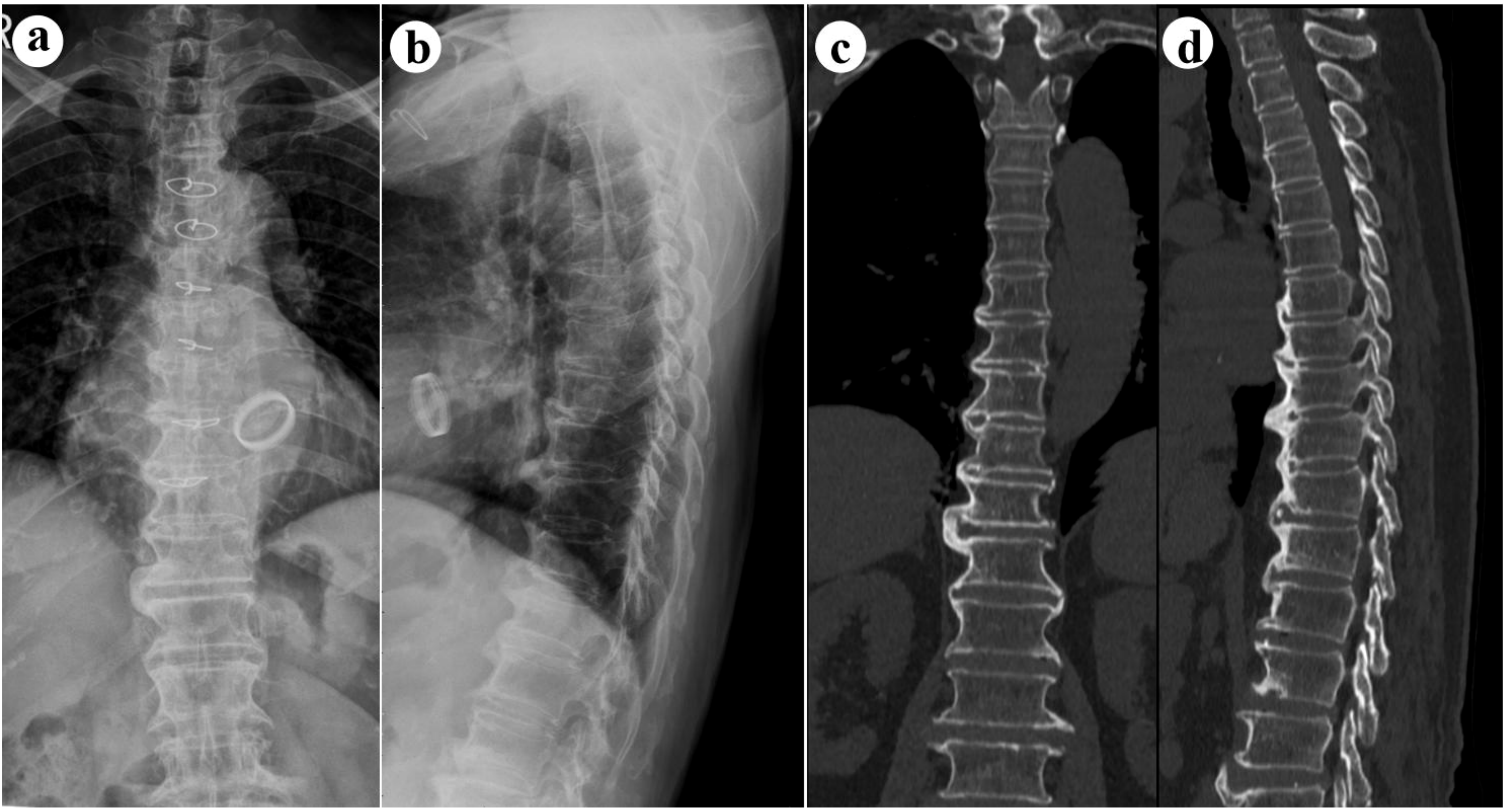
The characteristic radiological features of DISH. (a) An anterior-posterior view radiograph displays right-sided continuous ossification across the thoracic spine; (b) A lateral view radiograph reveals the presence of bony bridges anterior to the thoracic spine; (c) A coronal CT image depicts right-sided continuous ossification of the thoracic spine; (d) A sagittal CT image showcases bony bridges located in front of the thoracic spine. DISH indicates Diffuse Idiopathic Skeletal Hyperostosis; CT indicates Computed Tomography.
